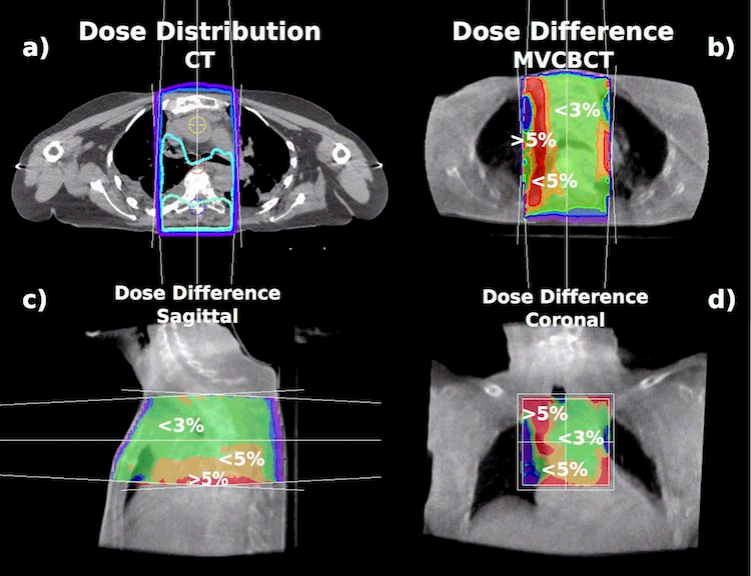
Dose difference for a thoracic spine plan: (a) dose distribution of a thoracic spine treatment with opposed AP/PA beams, planned on a standard CT. Relative dose difference planned on a 13.5 MU exposure eFOV MV CBCT in the transverse (b), sagittal (c), and coronal (d) views.
